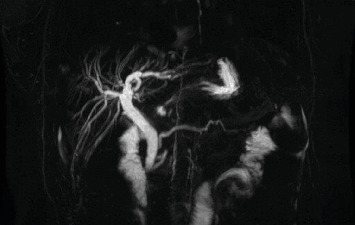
Magnetic resonance imaging (MRI) suspecting a potentially resectable distal cholangiocarcinoma.
